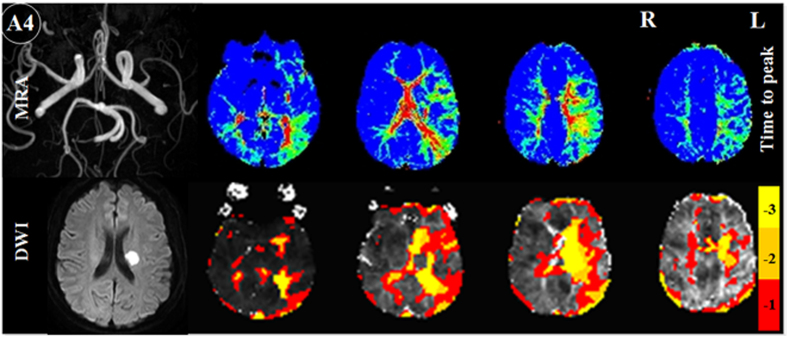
Perfusion injury detected by time to peak (TTP) on dynamic susceptibility-weighted contrast-enhanced perfusion images (top right) and TSA on resting-state functional MR images (bottom right) in subacute stroke patient with obvious thinness along the whole course compared to the right MCA. TTP delay was found in the left MCA corresponding blood supply area. TSA also demonstrated pronounced comparable time delay areas. MRA = magnetic resonance angiography, DWI = diffusion weighted imaging. −1, −2, −3 in color bar indicate −1TR, −2TR, −3 TR time shift.
